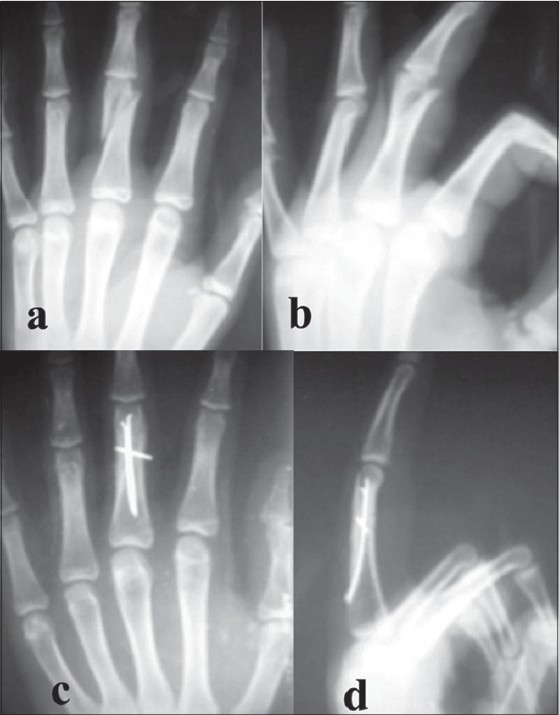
(a) Antero-posterior (AP) and (b) lateral view of right hand shows unstable long oblique fracture. AP and lateral X-ray (c and d) of right hand of the same patient shows additional interfragmentary K-wire for additional stability in long oblique fracture
